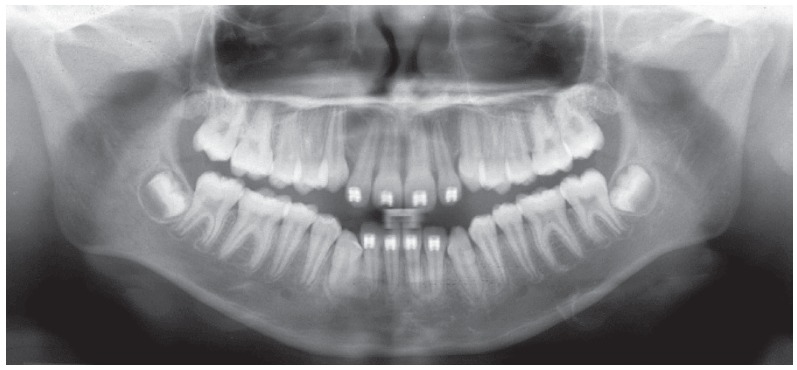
Intermediate panoramic radiograph.
